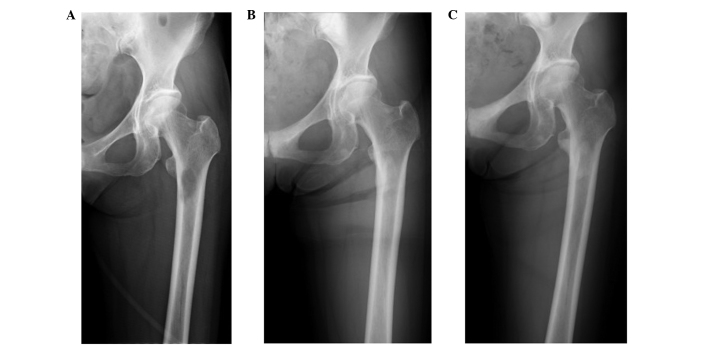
Plain radiography images showing changes in the metastatic site of the left femoral neck at (A) pretreatment, (B) 13 months following ZA initiation and (C) 21 months following ZA initiation. ZA, zoledronic acid.
